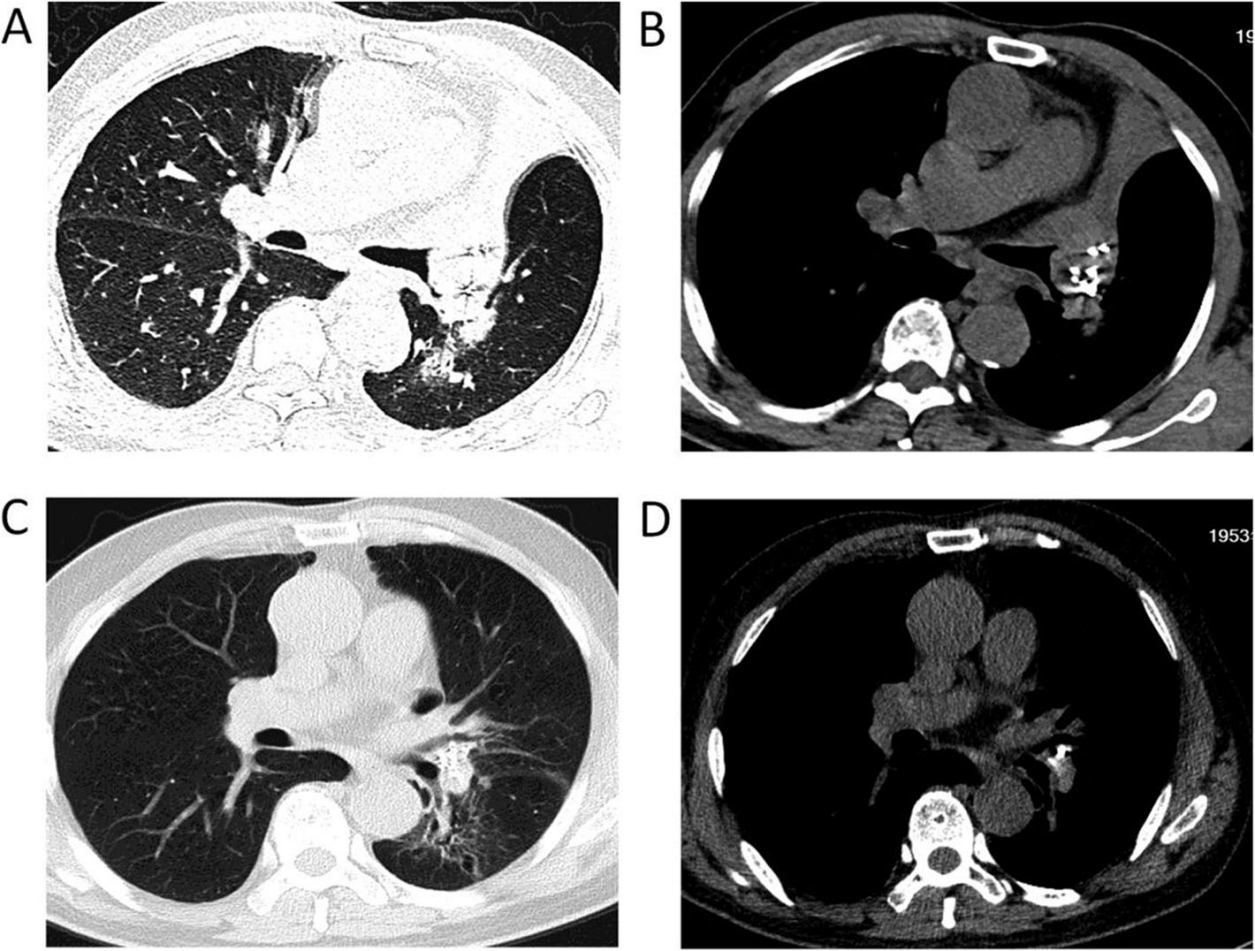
a, b Post-operative CT 3 days later confirmed that high-density ^125^I radioactive seeds were observed in the tumour (a: lung window, b: mediastinal window). c, d Compared with the previous chest CT scan, the chest CT scan at the second cycle after chemotherapy revealed disappearance of atelectasis and obstructive pneumonia in the left upper lung, as well as evident improvements in the lesions of the left hilum (a: lung window, b: mediastinal window)
